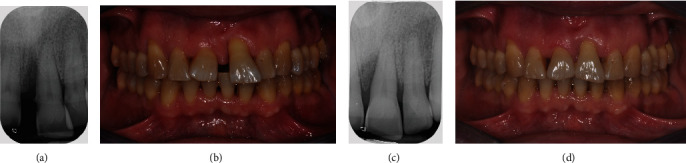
(a–d) Clinical and radiographic differences pre- and immediately postorthodontic treatment.
